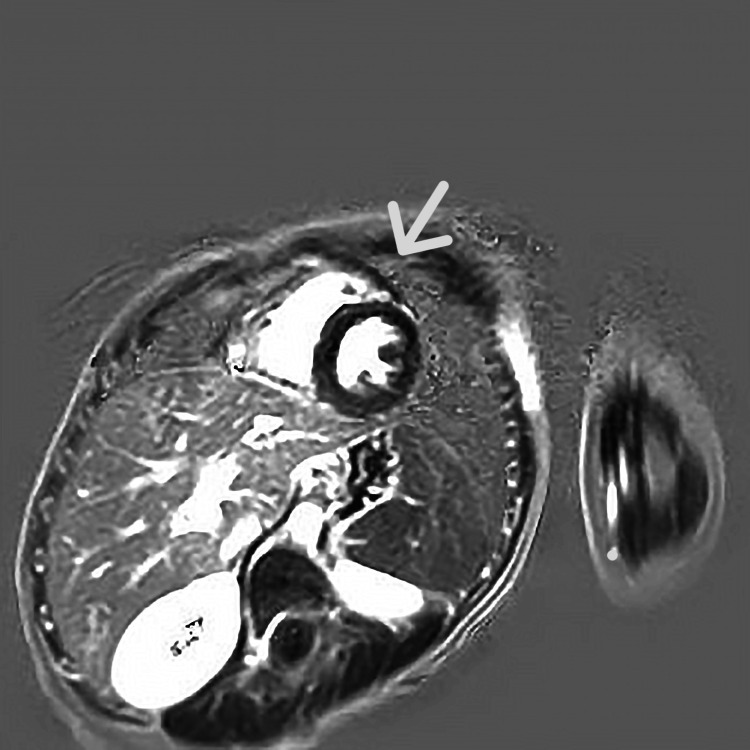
cMRI: Linear hypoechoic enhancement in the basal anterior and basal posterior septal wall of the left ventricle. cMRI: Cardiac magnetic resonance imaging
